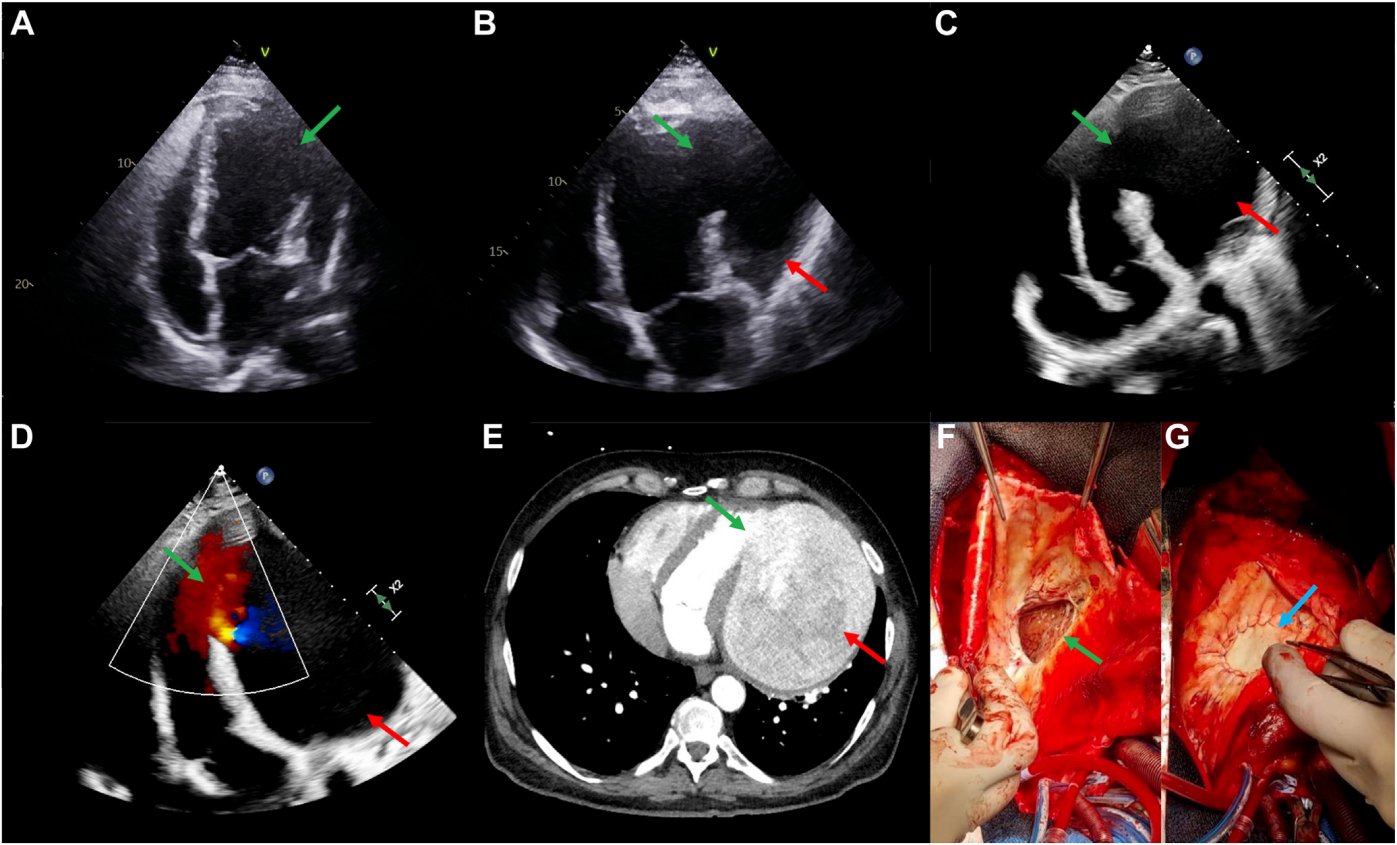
Left ventricular wall rupture (green arrows) and a pseudoaneurysm (red arrows) are shown on a transthoracic apical 4-chamber view (A) at the time of myocardial infarction, (B) 4 months later, and (C) 6 months later. (D) Colour flow Doppler echocardiography shows flow between the left ventricle and the pseudoaneurysm. (E) Cardiac computed tomography at 6 months shows the left ventricular wall rupture with a massive pseudoaneurysm. Intraoperative photography shows (F) the defect and (G) patch repair (blue arrow).
